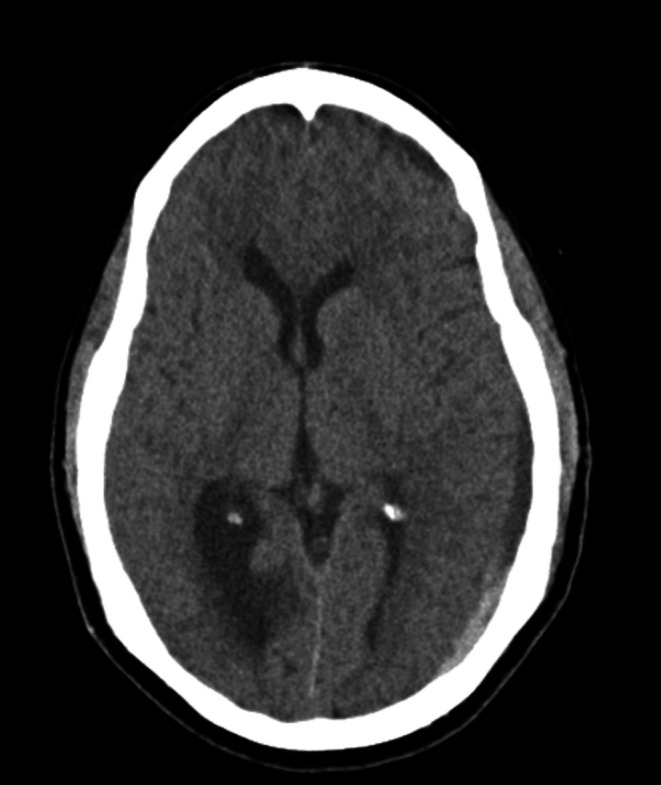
Post‐operative CT axial scan at post‐operative Day 1 showing interval decrease in size of the left subdural hematoma with associated decrease in mass effect and subfalcine herniation. Stable postsurgical changes following a burr hole for subdural evacuation.
